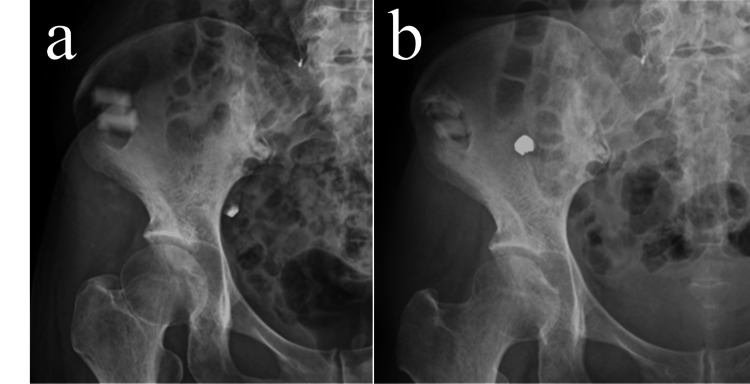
Plain radiographs of the donor site. (a) Immediately after surgery. (b) Two years postoperatively.
